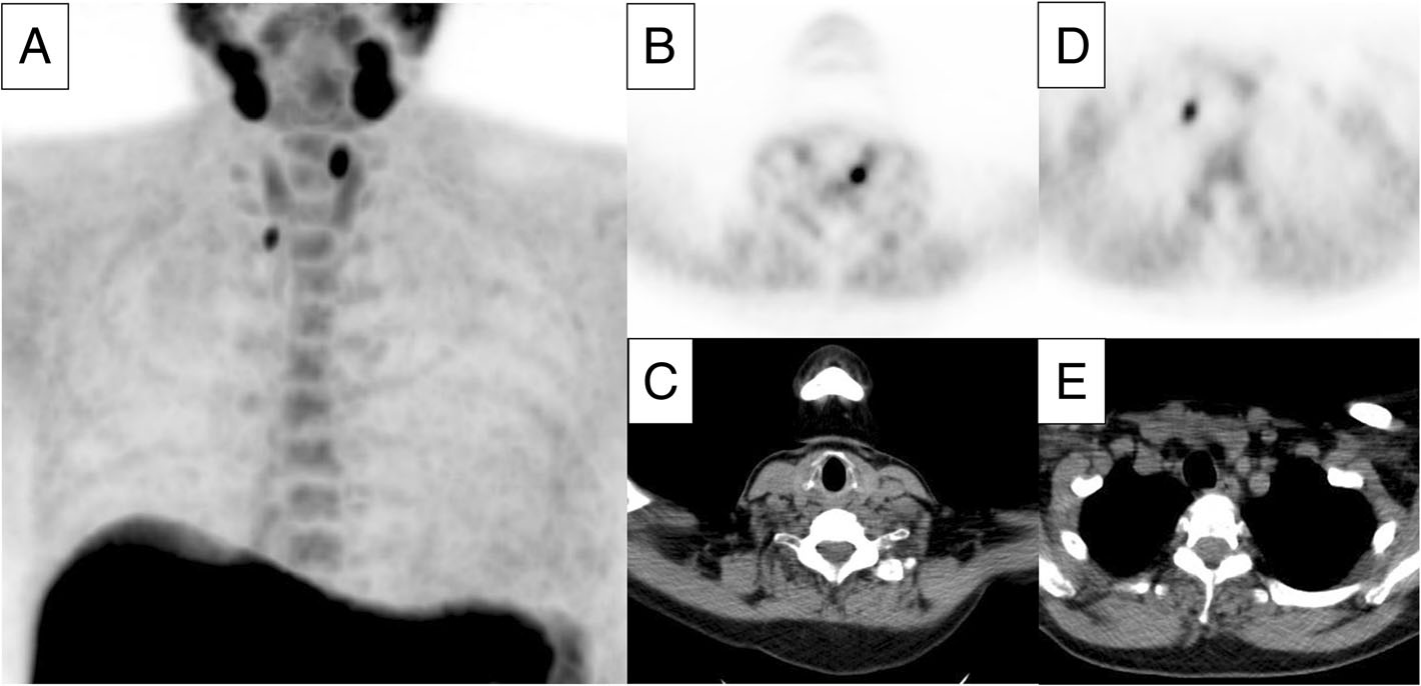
Example of a positive ^18^F-fluorocholine PET/CT representing a double parathyroid adenoma. Serum calcium level in this patient was 2.61 mmol/L with a parathyroid hormone level of 27.7 pmol/L. The maximum intensity projection image (a) shows two foci of intense choline uptake in the neck and physiological uptake in the salivary glands, thyroid gland, liver, spleen, and bone marrow. Axial views of PET and CT show foci suspicious for parathyroid adenomas located dorsocranial to the left thyroid lobe (b, c, dimensions 7 × 5 mm and SUV_max_ of 8.7) and inferior to the right thyroid lobe (d, e, dimensions 6 × 4 mm and SUV_max_ of 5.6)
